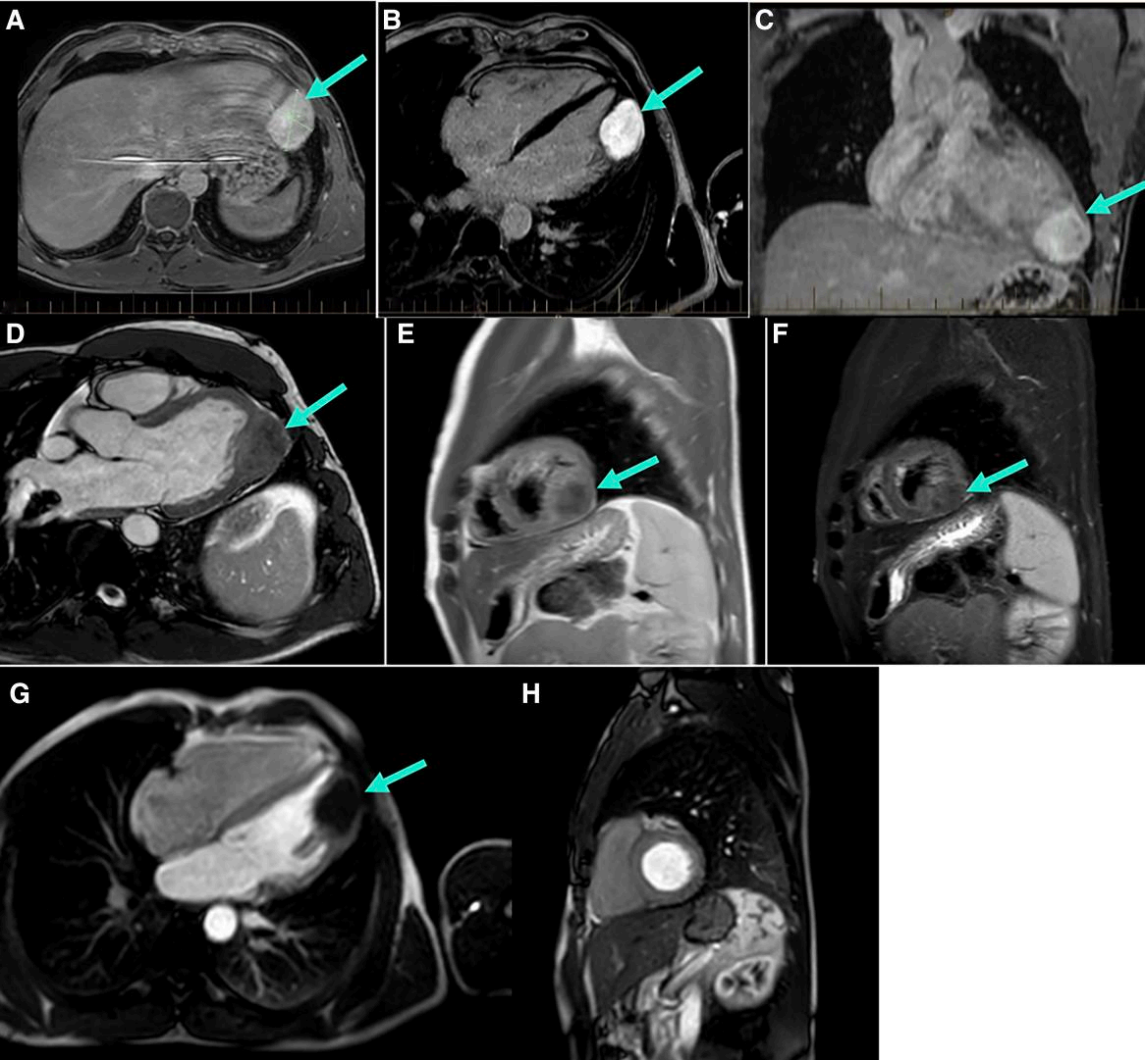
Cardiac magnetic resonance imaging demonstrating the morphological and tissue characteristics of the left ventricular mass. Axial (*A* and *B*) and coronal (*C*) late gadolinium enhancement images show a well-circumscribed intramyocardial mass with intense, homogeneous delayed enhancement (blue arrows). T1-weighted (*D* and *E*) images show the lesion as isointense to mildly hypointense relative to normal myocardium, while T2-weighted short tau inversion recovery imaging (*F*) demonstrates low T2 signal intensity, consistent with dense fibrous tissue. First-pass perfusion sequences (G: axial; H: sagittal) show absent enhancement within the mass, confirming its avascular nature. The overall pattern of low T2 signal, homogeneous intense late gadolinium enhancement, and absent first-pass perfusion is characteristic of a cardiac fibroma, distinguishing it from other cardiac tumours such as myxoma, rhabdomyoma, or sarcoma.
